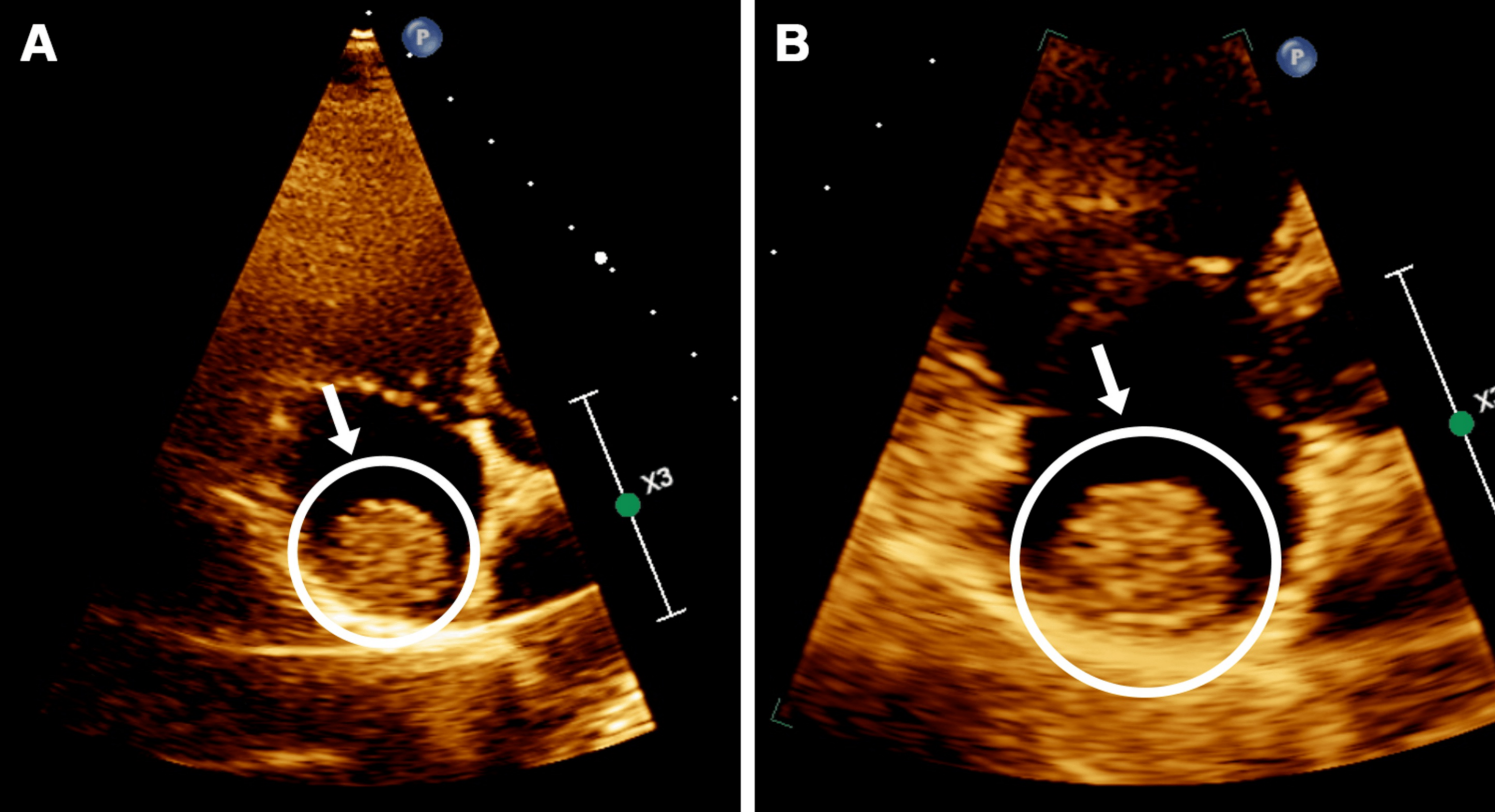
Echocardiogram image of the right atrial myxoma before surgical removal (August 2020)
